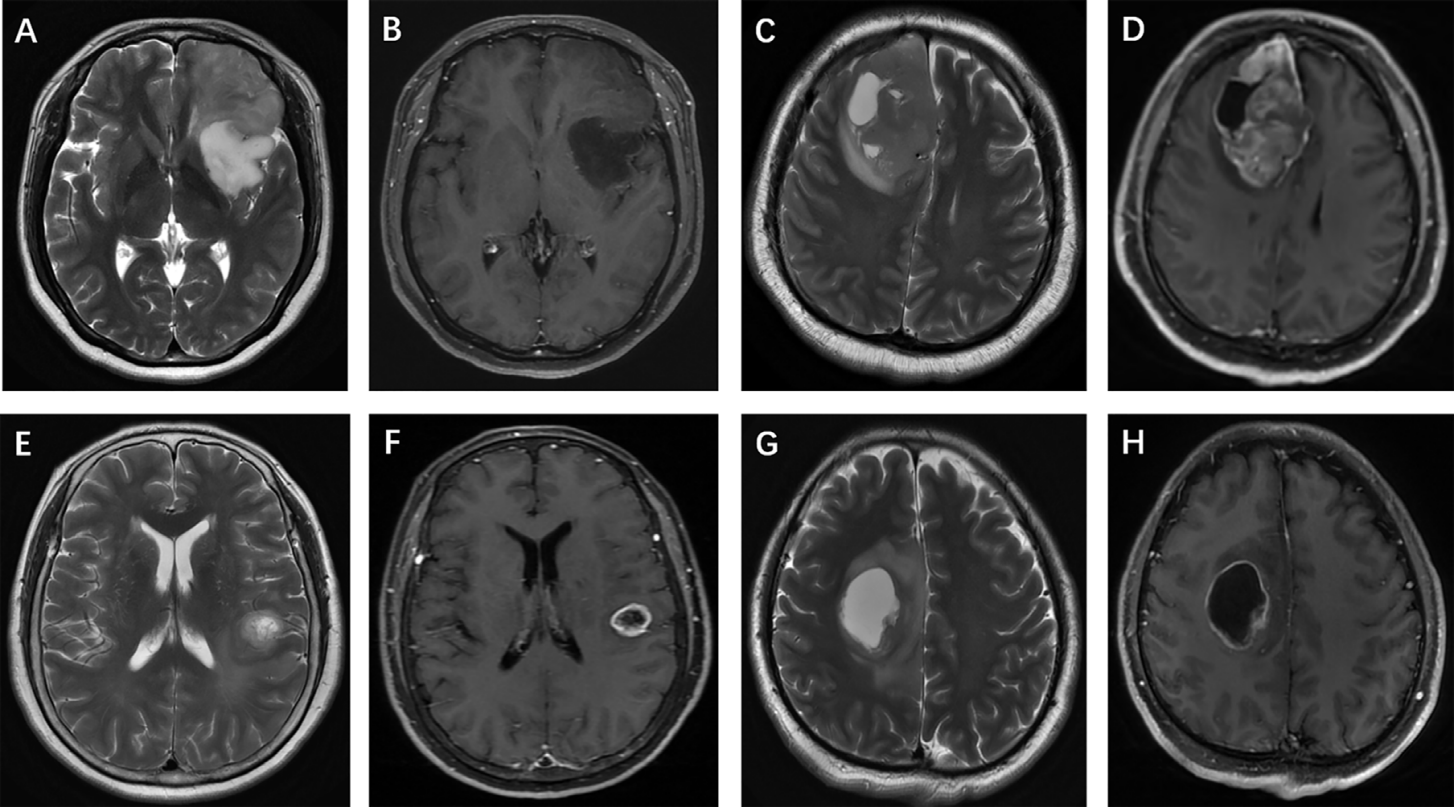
Glioma morphologic characteristics. Enhancement pattern: (A, B) T2-weighted imaging shows a left frontal isocitrate dehydrogenase (IDH)-mutant oligodendroglioma without contrast agent uptake; (C, D) T2-weighted imaging shows a right frontal IDH-wildtype glioblastoma, and contrast-enhanced T1-weighted imaging shows patchy contrast uptake; (E, F) T2-weighted imaging and contrast-enhanced T1-weighted imaging show rim enhancement surrounding a central necrosis in a IDH-wildtype astrocytoma, while another patient (G, H) presents with a frontal IDH-mutant glioblastoma.
